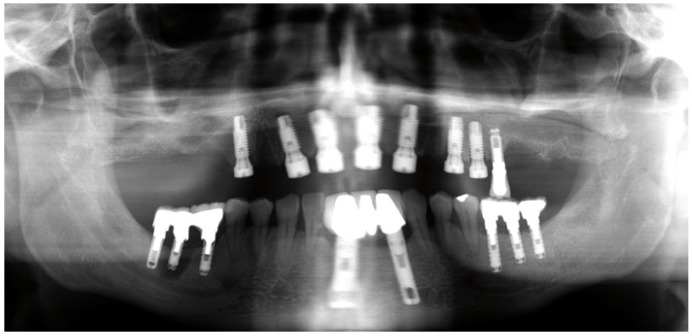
Case 2. 7. Postoperative panoramic X-ray view after sinus lift with the balloon technique. Implant placement at this level was postponed three months due to an insufficient initial alveolar crest height (2.1 mm).
